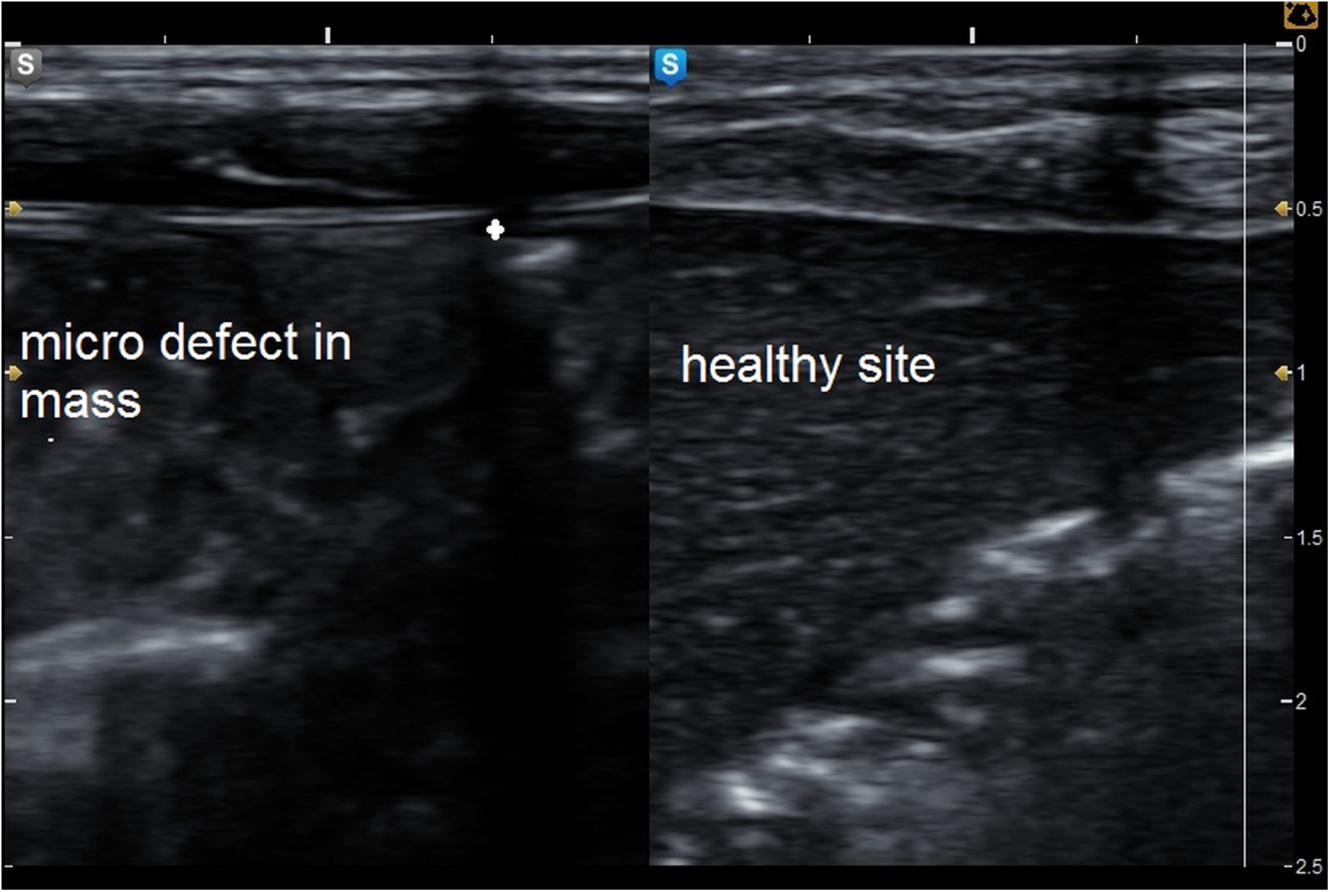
Grey mode ultrasound showed micro defect in the muscle sheath complex with comparison to the healthy side of the abdominal wall.
